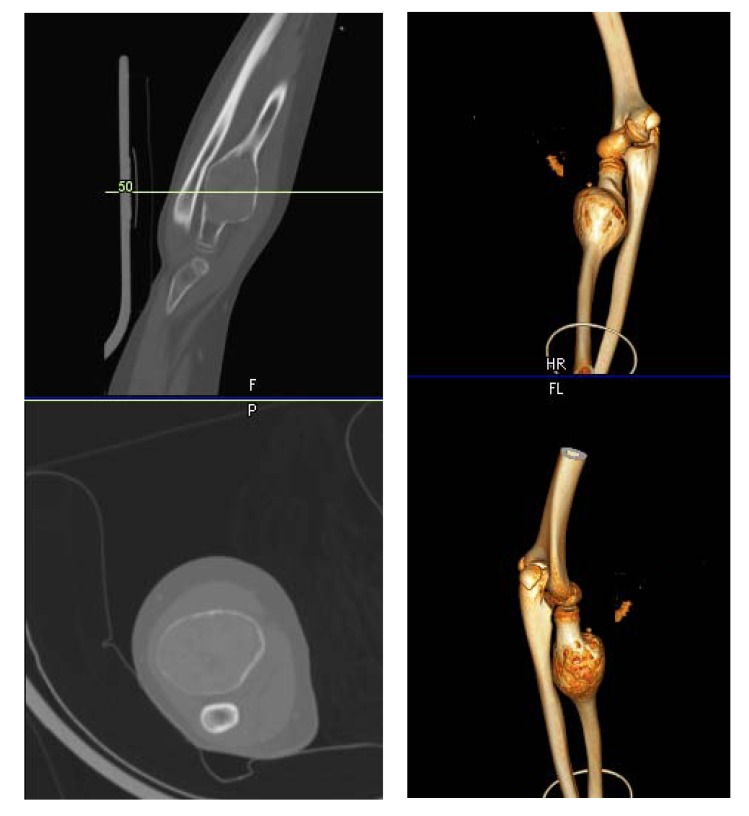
Pre-operative CT scan images showing markedly expansile lytic lesion measuring 4.5 cm X 3.8 cm in transverse diameter at proximal radial shaft with thinning of cortex, short trasitional zone, without periosteal reaction or surrounding soft tissue involvement.
